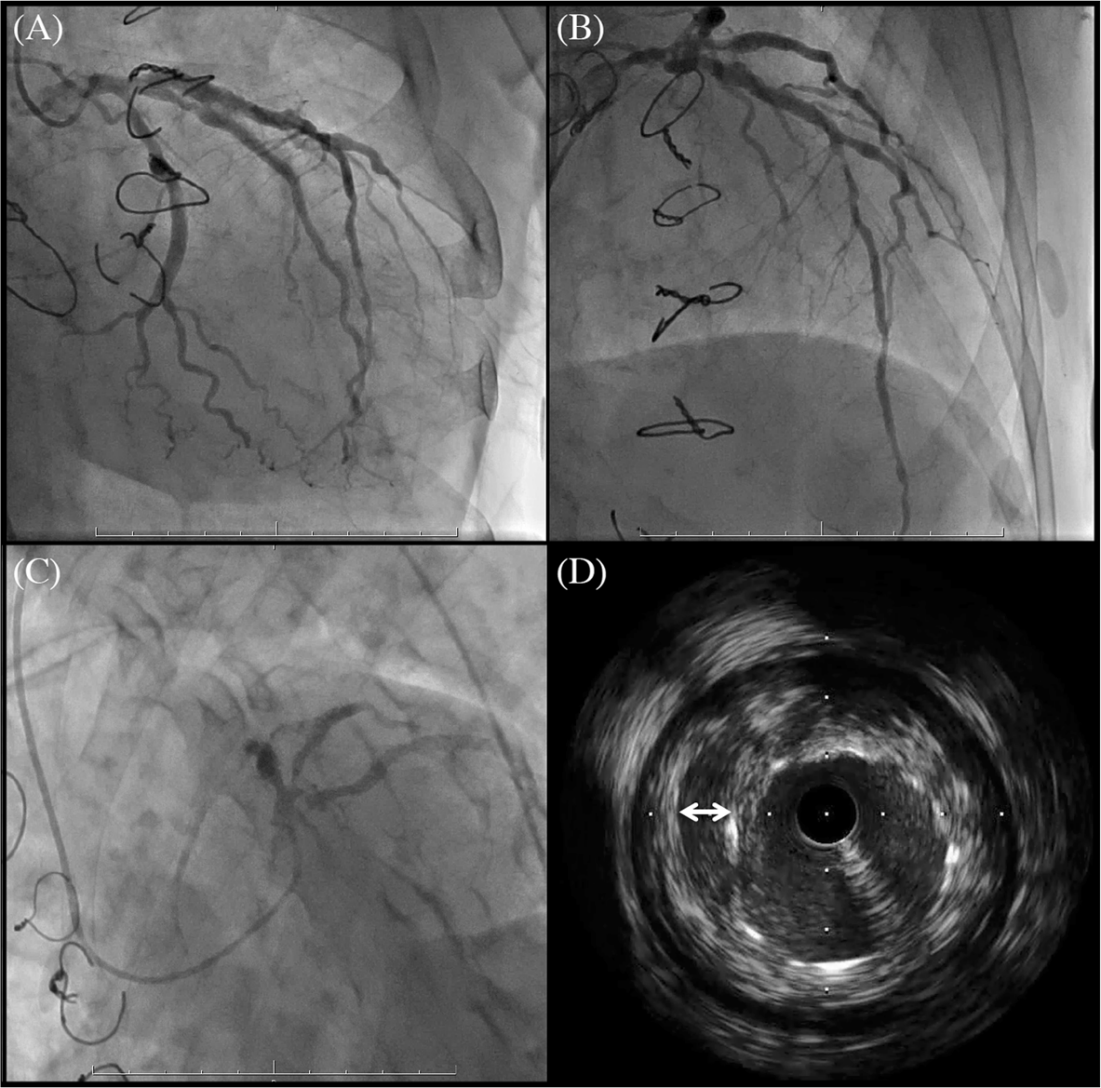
Preoperative coronary angiography (a–c) shows stenoses of the left anterior descending artery, high lateral branch, and left circumflex artery. Intravascular ultrasound (d) shows a stent malapposition at the site of the coronary aneurysm
